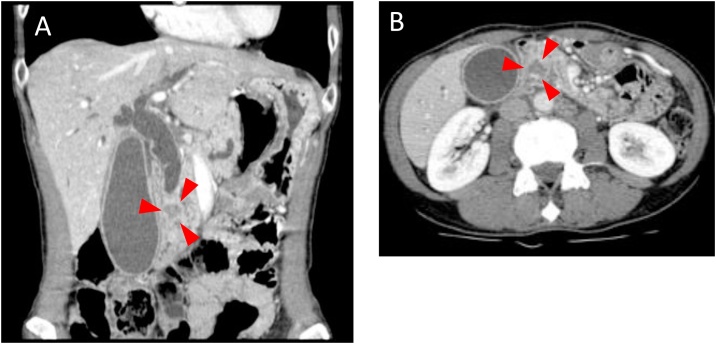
Computed tomography (CT) scan showing pancreatic head tumor (red arrow heads) causing dilatation of biliary and pancreatic ducts. A: axial view; B: coronal view.
